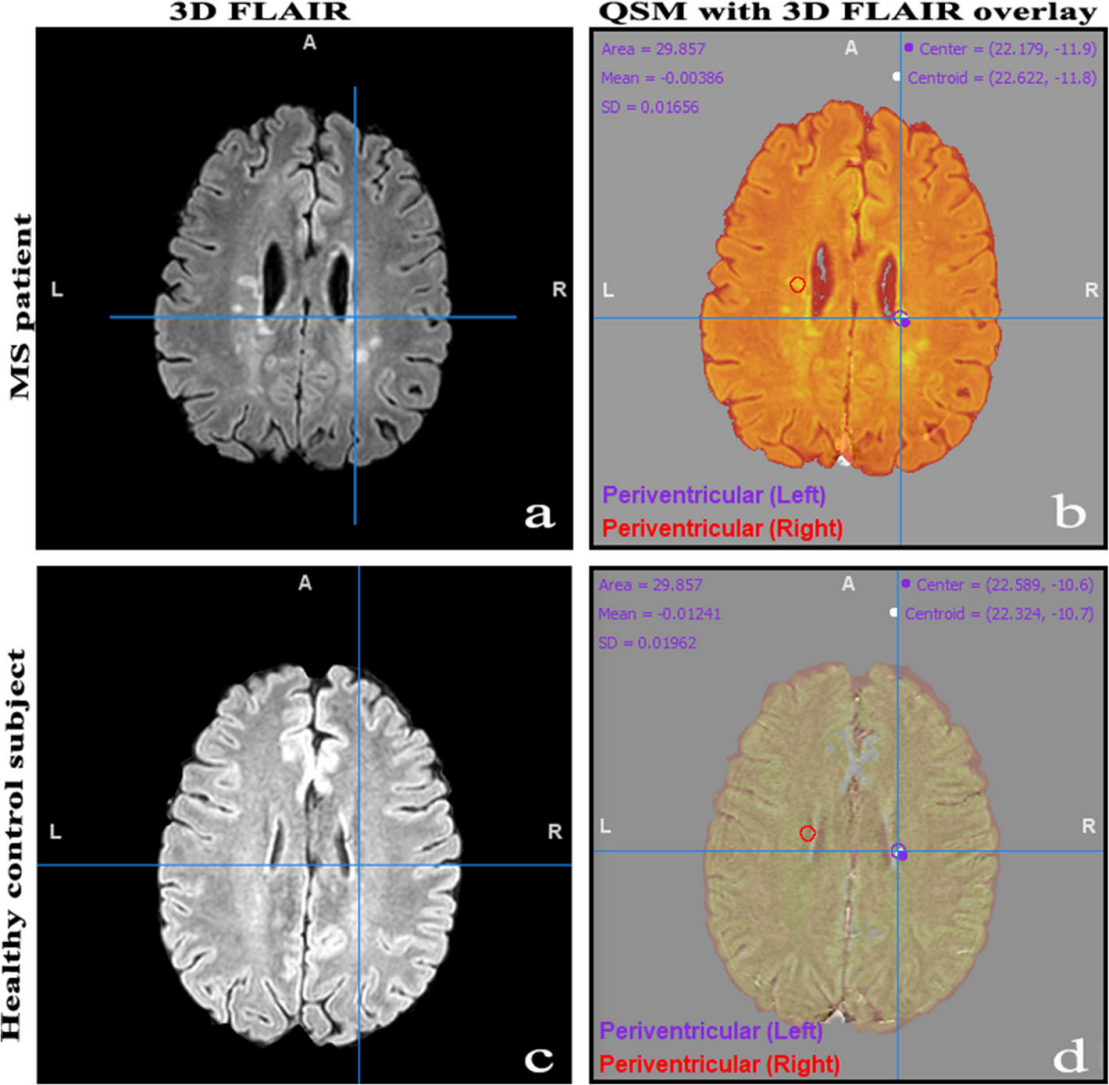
Measuring regional iron load in a 39-year-old man with relapsing-remitting multiple sclerosis (A, B) in periventricular lesions and measuring iron load in corresponding locations in a healthy 44-year-old male control subject. A represents an axial three-dimensional fluid-attenuation inversion recovery (3D FLAIR) film of the patient demonstrating two periventricular lesions (right and left). The 3D FLAIR film was overlayed on the quantitative susceptibility map as depicted in image B and the transparency was set to 50% to visualise both the quantitative susceptibility mapping (QSM) and the 3D FLAIR images. Two regions of interest (ROIs), shown as red and violet circles, were placed in the periventricular lesions and the ROIs were analysed on the QSM image. C Represents a corresponding axial 3D FLAIR cut of a healthy man. Similar steps were performed to measure iron in approximate locations corresponding to the MS periventricular lesions
